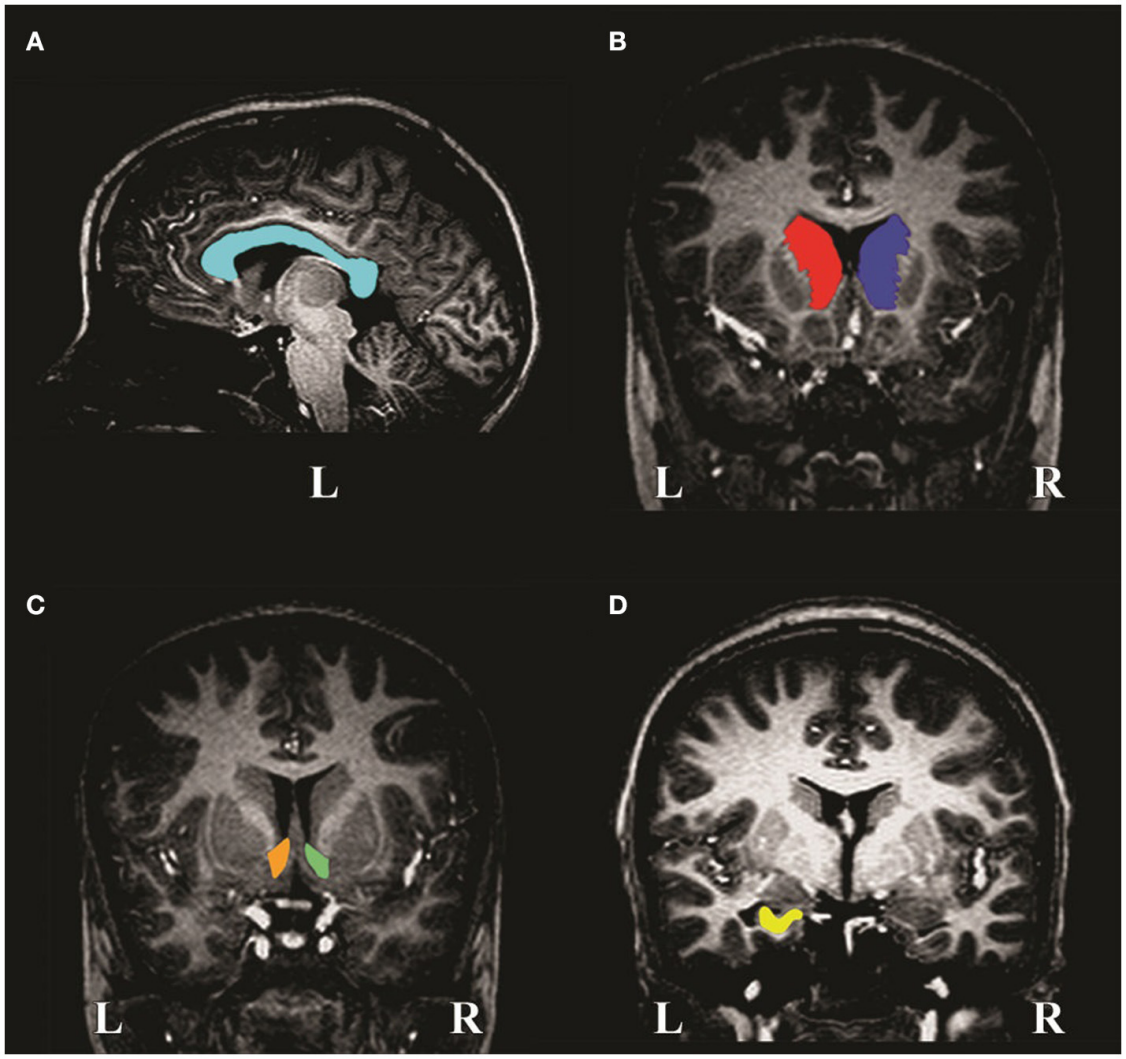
MR image slices of a non-exposed control child showing manual tracings of the (A) corpus callosum, (B) left and right caudate nuclei, (C) left and right nucleus accumbens and (D) left hippocampus. L and R denote left and right, respectively.
